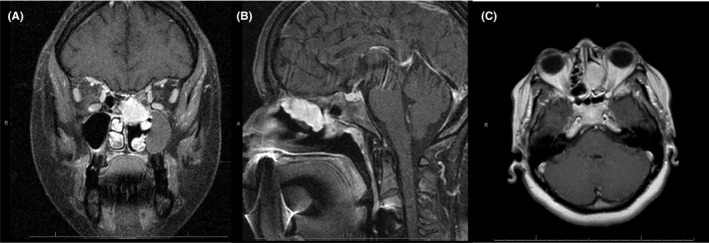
A‐C, MRI at diagnosis. The 2.1 × 1.7 × 3.5 cm mass in the superior left nasal cavity demonstrates avid enhancement. There is no MRI evidence of perineural tumor extension, intracranial extension into the cribriform plate, or cavernous sinus involvement
